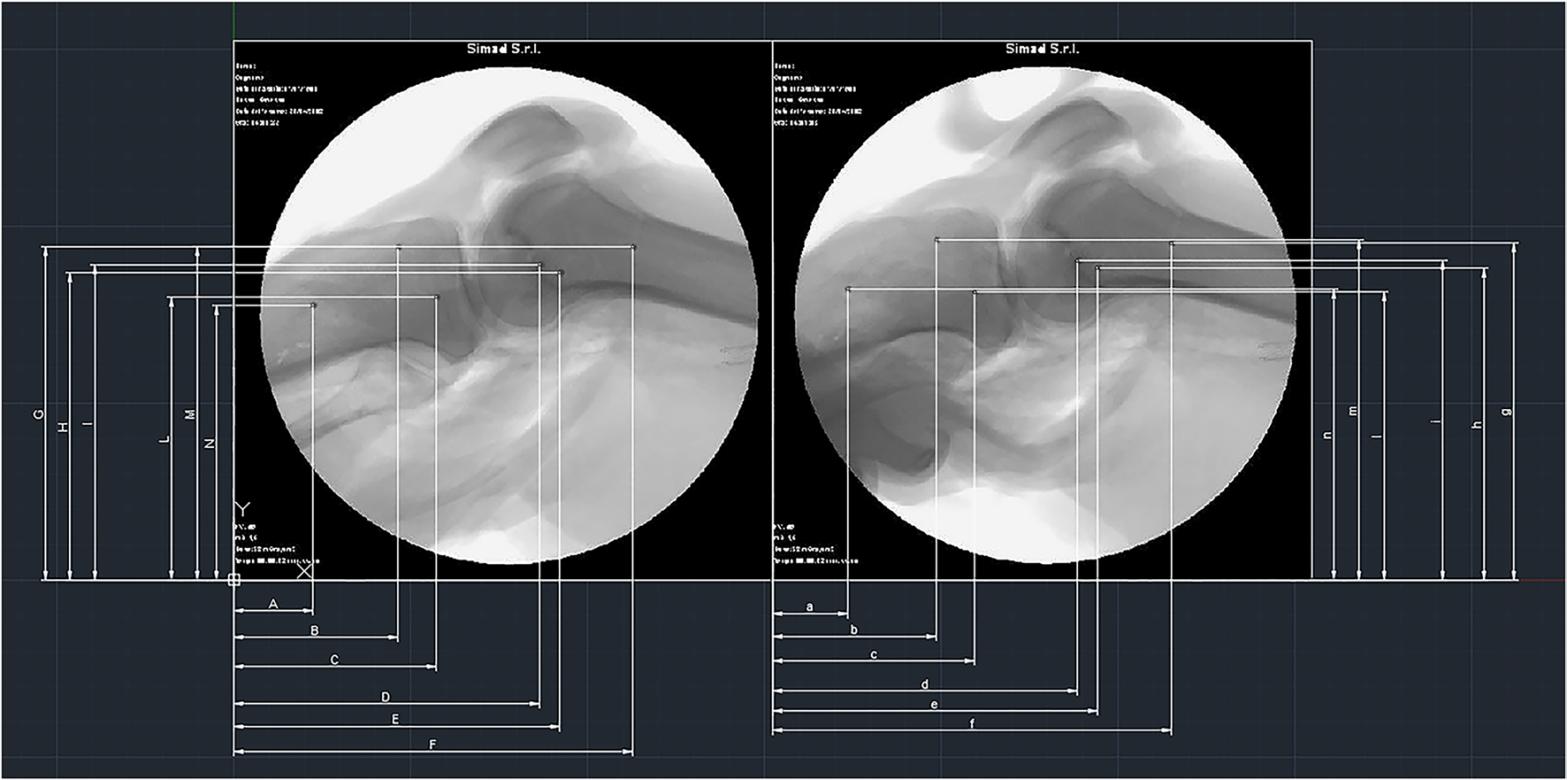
Example of the method used to obtain objective ATT data by stress radiographs, by tracking the differences between the initial position of the tibia (resting phase, RP) and its final position during testing (loading phase, LP). For each of the 6 titanium spheres identified within the radiograph, an X- and Y-coordinate was identified and the X- and Y-components of the cluster of 3 titanium spheres of the tibia and femur were averaged to obtain the exact difference (delta, Δ) of tibial and femoral position between the RP and LP
